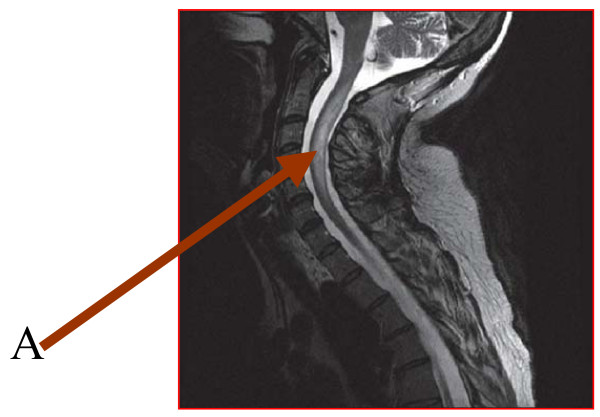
Cervical spine magnetic resonance imaging showing the hyperintense focus (Arrow A).
